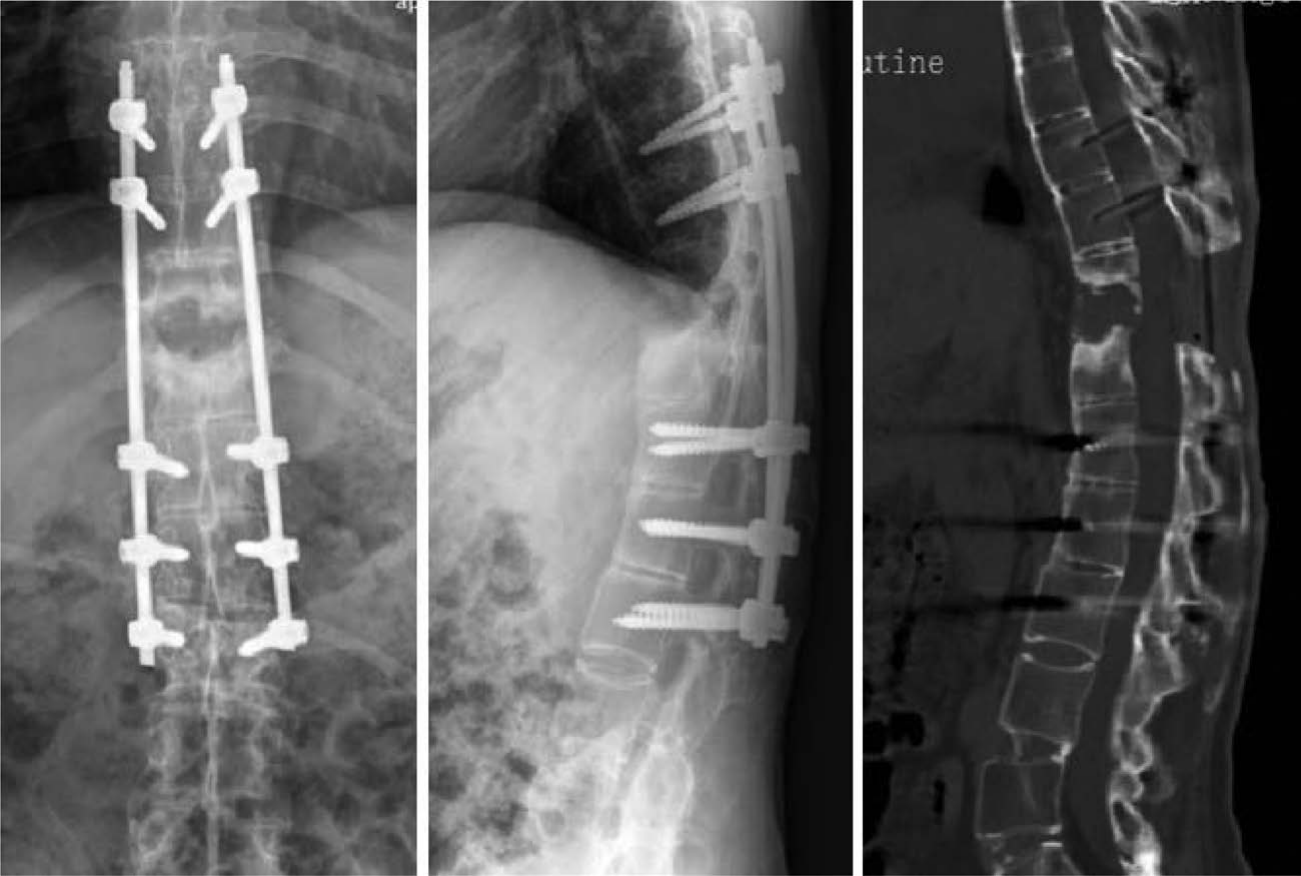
The radiograph and CT scan of the initial surgery demonstrate complete decompression, proper positioning of the internal fixation, and a significant anterior gap created by posterior fixation to correct the kyphosis. CT = computed tomography.
